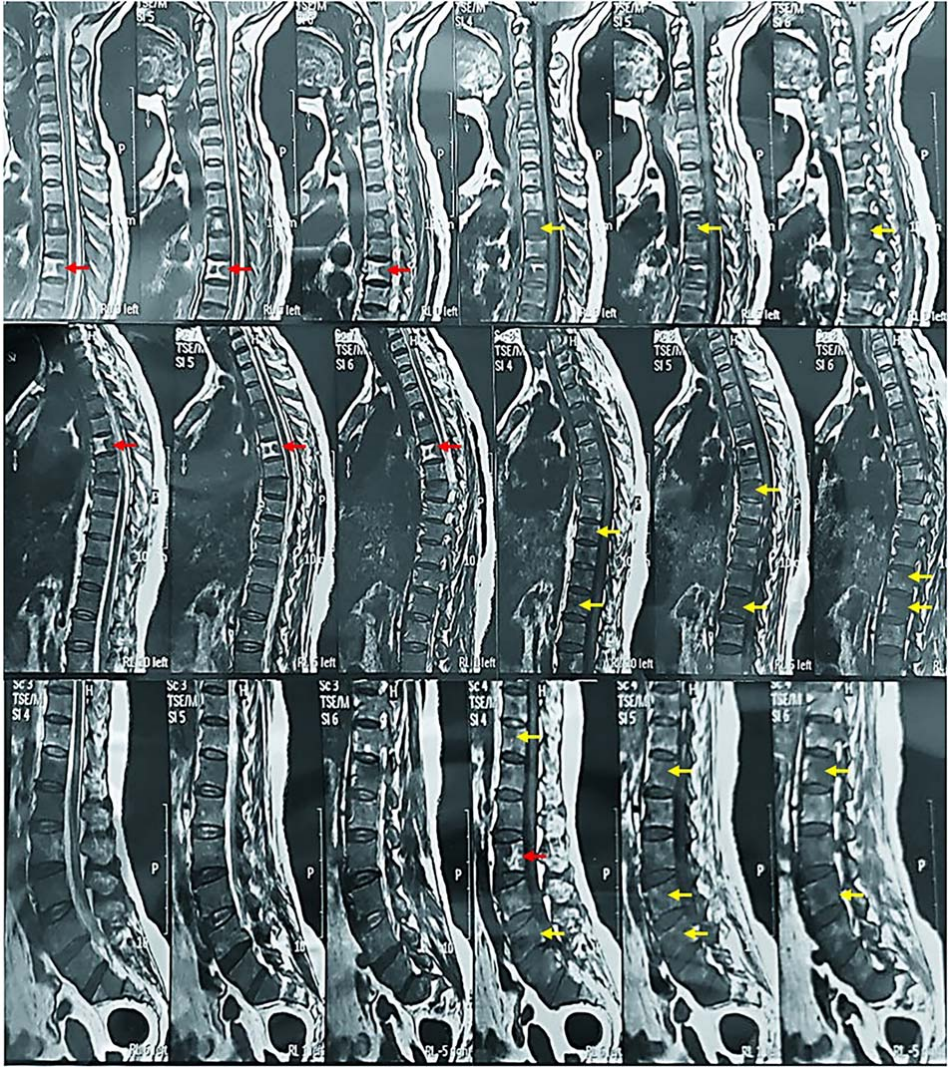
The patient’s MRI showed diffuse lytic (yellow arrow) and sclerotic (red arrow) lesions in the patient’s vertebras.
